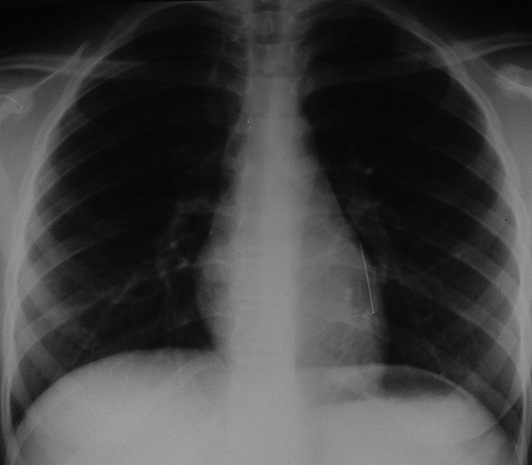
Radiographie thoracique de face: épingle à foulard se projetant sur la lobaire inférieure gauche
